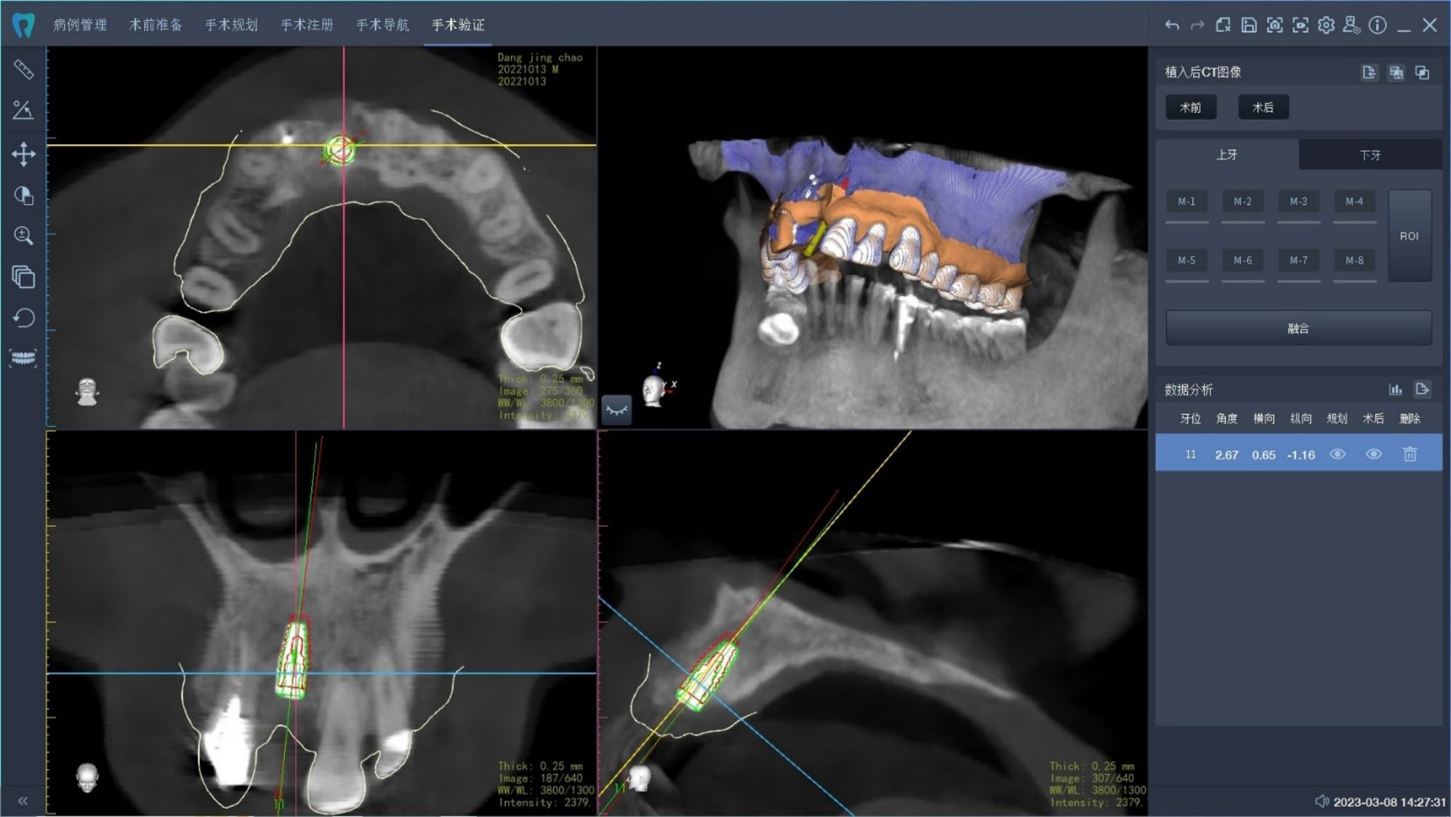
Superimposition of the postoperative CBCT and the preoperative plan showing the planned implant (red) and the actual implant outlines (green)
